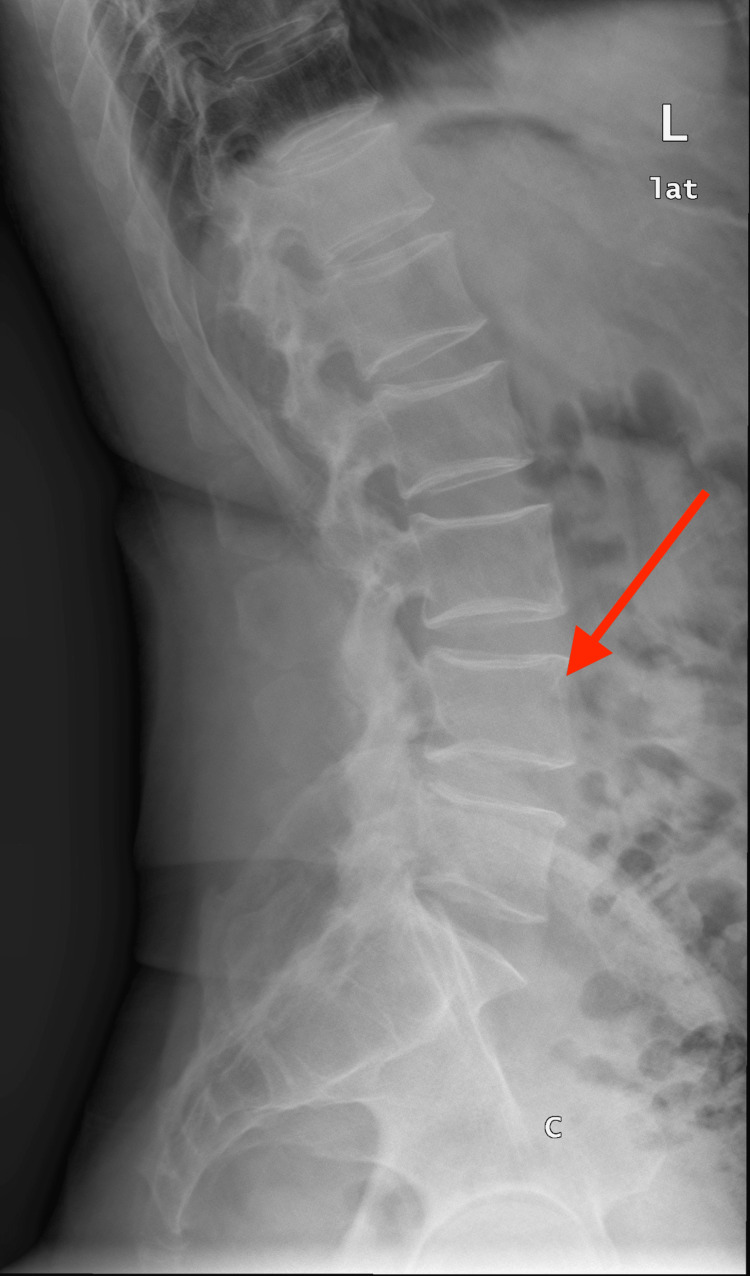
X-ray of the lumbar spine showing degenerative disc changes at the lower lumbar discs Red arrow: degenerative changes in the lower lumbar discs
